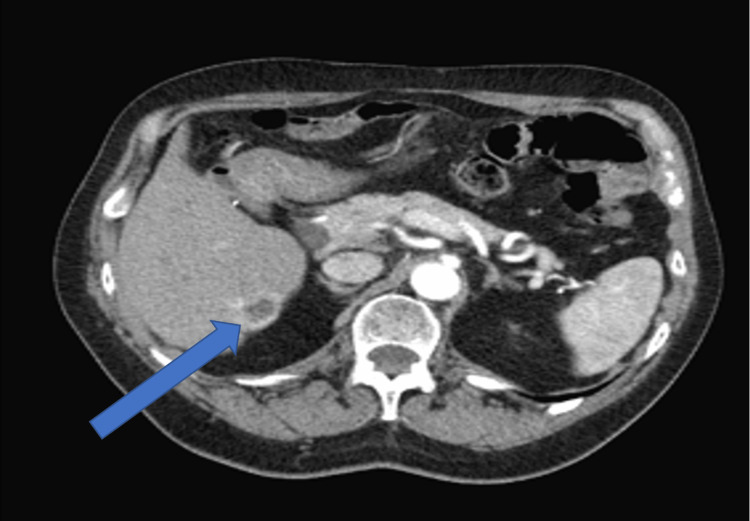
CT abdomen and pelvis triple-transverse view phase demonstrating a 2.6 -centimeter lesion in liver segment 6
